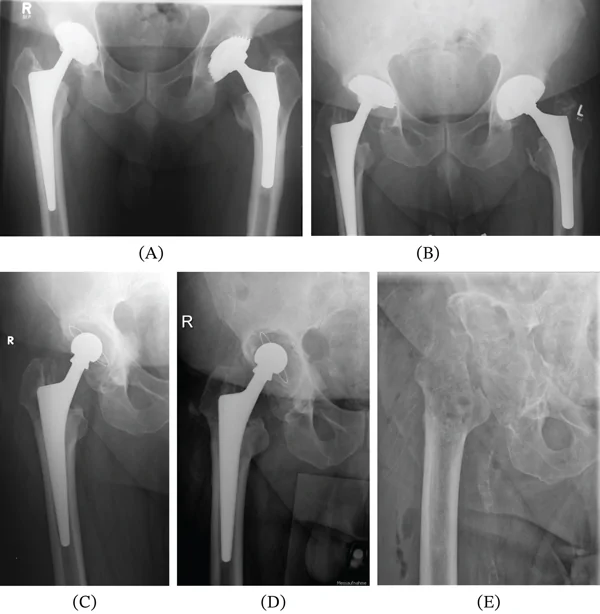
(A) Anteroposterior pelvic radiographs obtained in 2001. (B) Anteroposterior pelvic radiographs obtained in 2009 with cup loosening on the right side and performed cup revision on the left. (C) Anteroposterior pelvic radiographs obtained in 2011. (D) Anteroposterior pelvic radiographs obtained in 2014 with gradual loosening of the Slooff and increasing cup inclination. (E) Anteroposterior pelvic radiographs obtained in 2024 after explantation and conversion to a Girdlestone situation.
